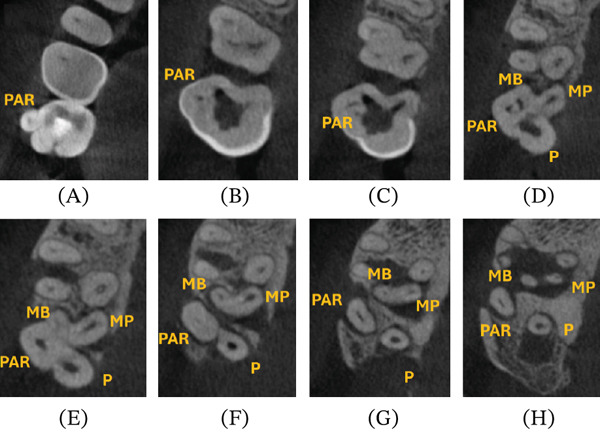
Tooth #2 axial views in CBCT (PAR: parastyle; MB: mesiobuccal; MP: mesiopalatal; P: palatal). (A–C) Clinical crown level. (D) Coronal root third. (E, F) Midroot third, (G, H) Apical root third.
